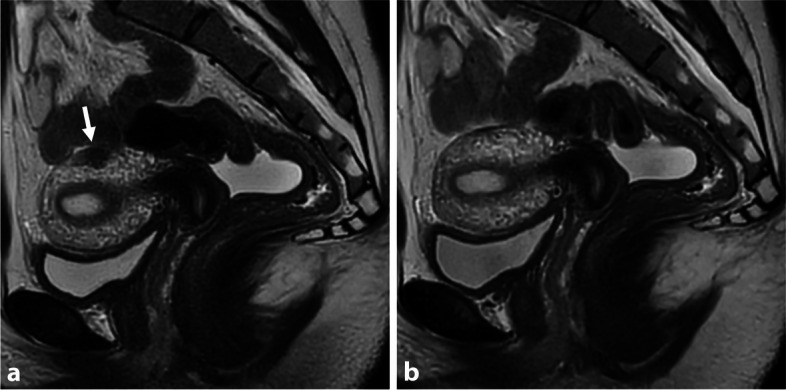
Uterine contraction in a 19-year-old woman with chronic catamenial pelvic pain. a Sagittal T2-W MR image shows a T2-hypointense focal thickening (arrow) of the outer myometrium on the back wall of the uterus. b Note the absence of abnormality of the myometrium and its complete resolution on additional T2-W MR sequences repeated at the end of the exam
